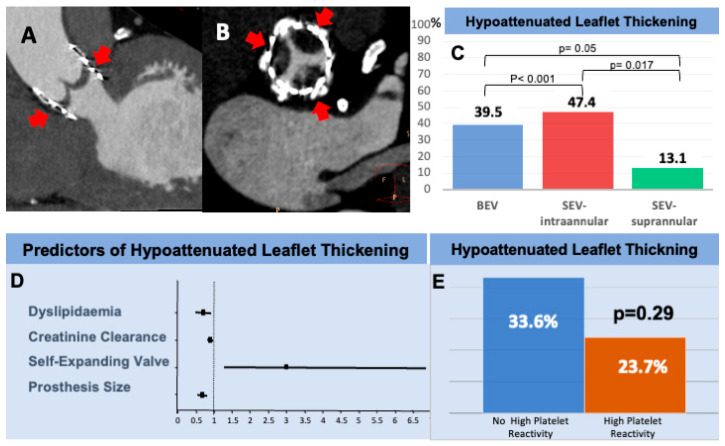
The occurrence of HALT according to valve type. (A,B): An example of a computed tomography image performed 3 months after transcatheter valve replacement in a patient with hypoattenuated leaflet thickening in the three cusps (red arrows). (A): Long-axis view; (B): short-axis view. (C): Incidence of hypoattenuated leaflet thickening by valve type implanted. BEV: Balloon-expandable valve; SEV: self-expanding valve. (D): Predictors of hypoattenuated leaflet thickening. (E): Incidence of subclinical leaflet thrombosis in patients with high residual platelet reactivity compared with patients without high residual platelet reactivity.
